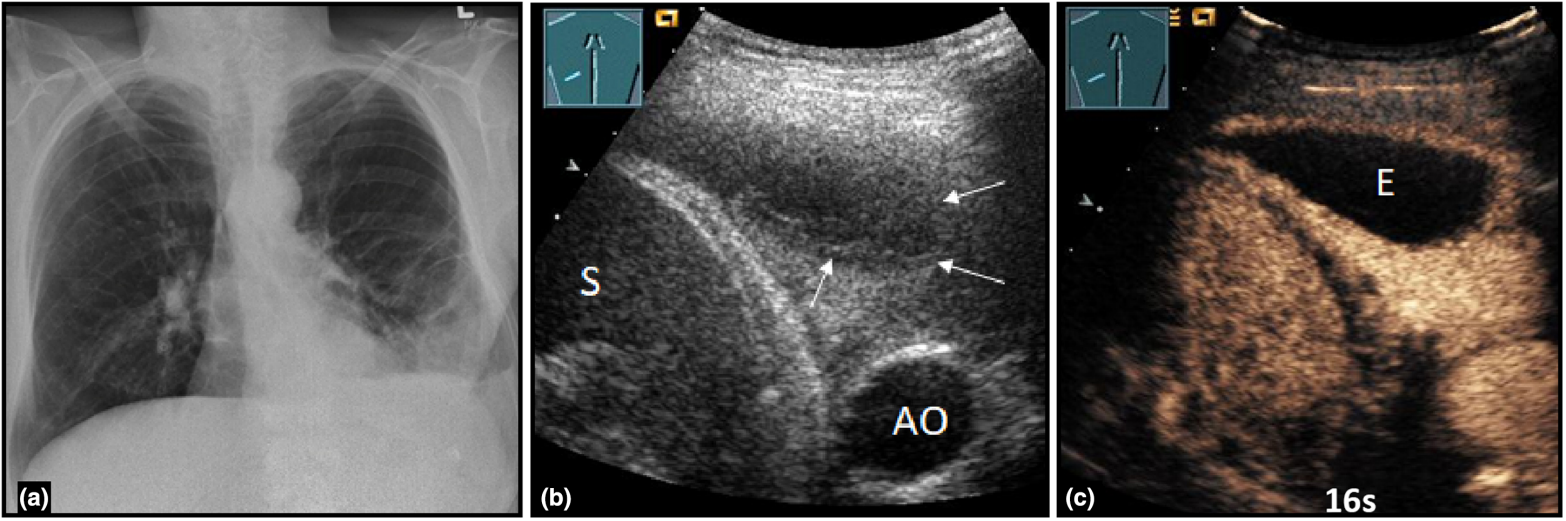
A 62‐year‐old female patient presented to the emergency department with shortness of breath and left‐sided flank pain. Presentation of a left‐sided subfield consolidation on the thoracic X‐ray (courtesy of Prof. Dr. Andreas H. Mahnken, Department of Radiology, University Hospital Marburg) (a); on B‐mode ultrasound (b), a hypoechoic consolidation with an oval, pleural, nearly anechoic area (arrows) is seen (AO = aorta, S = spleen); on contrast‐enhanced ultrasound (c), the consolidation shows a homogeneous perfusion pattern of enhancement after 16 seconds. Furthermore, a pleural‐based oval area with absent enhancement and marked wall enhancement is present, consistent with community‐acquired pneumonia with pleural empyema (E). A diagnostic/therapeutic thoracentesis of the empyema was performed.
